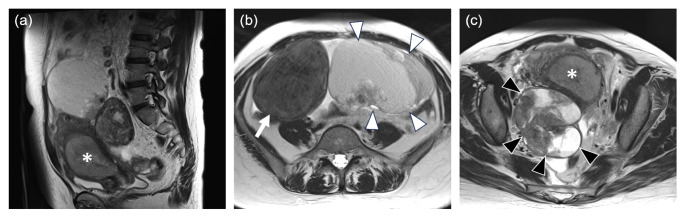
Pelvic MRI at Initial Presentation (T2-Weighted Images). (a) A mass (*) filled the uterine cavity with > 50% myometrial invasion.(b) The left ovarian tumor (white arrowhead) measured approximately 12 cm in maximal diameter and contained mixed cystic and solid components. A uterine leiomyoma (arrow) was also noted on the left side of the uterine fundus. (c) The right ovarian tumor (black arrowhead) measured approximately 12 cm in maximal diameter and contained mixed cystic and solid components. The intrauterine mass (*) was also visible on the same slice. MRI, magnetic resonance imaging
